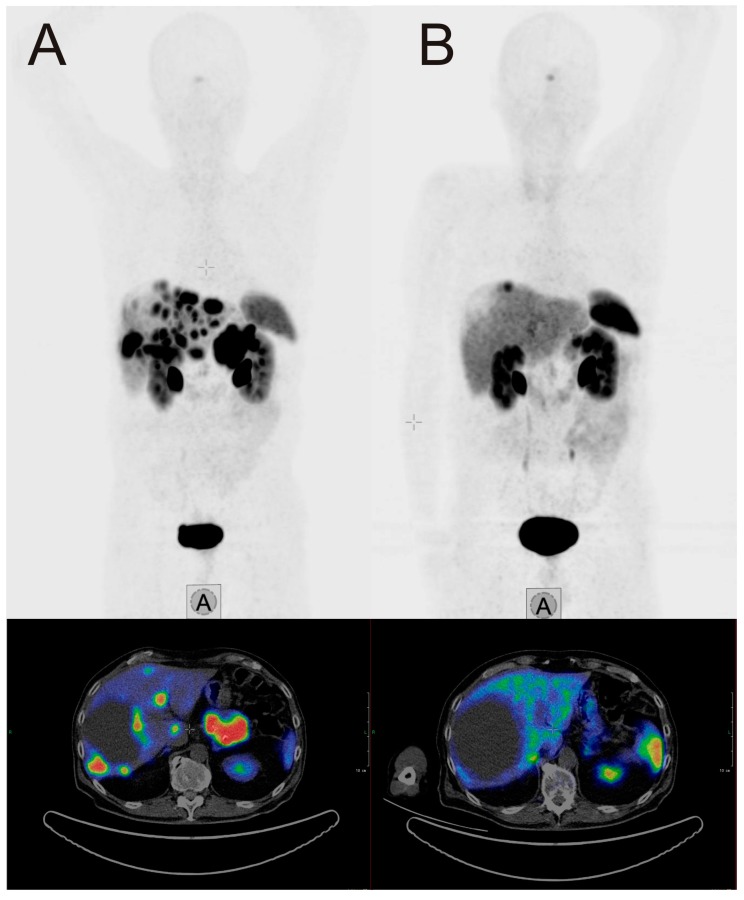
Example of a 70-year-old male patient with a pancreatic neuroendocrine tumor (NET), first diagnosed in April 2017, with an initial ki-67 index of 3%. After initial treatment with everolimus, liver metastases showed progress in November 2017. (A) SSTR-PET/CT at this time point. The patient was treated with four cycles ^177^Lu-DOTATATE (total dosage 29.3 GBq) between November 2017 and July 2018. Two months after the last cycle, SSTR-PET/CT (B) showed a very good response. Chromogranin dropped in the same interval from 1004 µg/L to 140 µg/L.
